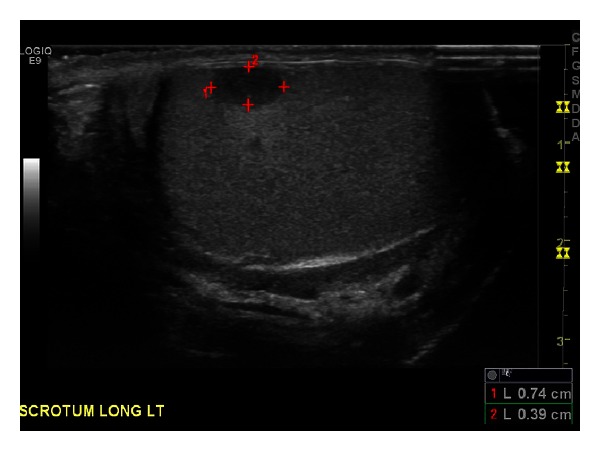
Scrotal ultrasound showed a hypervascular lesion of the left testicle consistent with malignancy.
